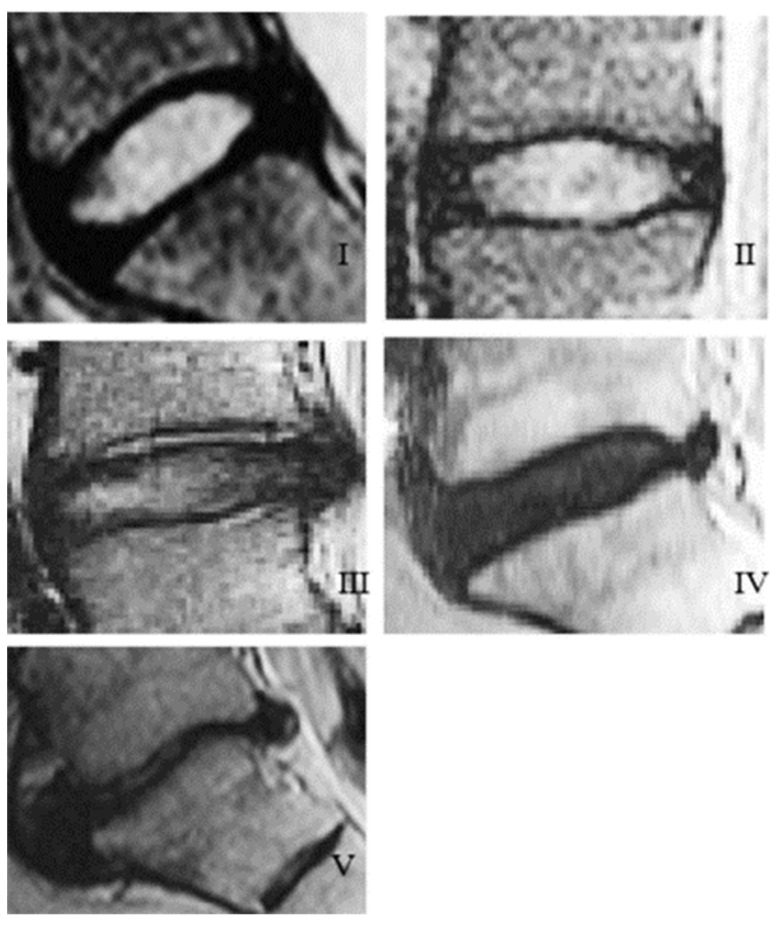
MRI scans showing the different grades of disc degeneration based on the Pfirrmann grading system, (I–V) referring to Grades (I–V): I is representative of Grade (I) degeneration, (II) is representative of Grade (II) degeneration, (III) is representative of Grade (III) degeneration, (IV) is representative of Grade (IV) degeneration, and (V) is representative of Grade (V) degeneration [88].
